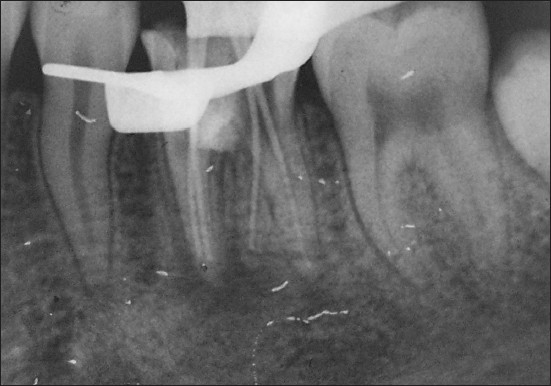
Master cone selection radiograph evidencing successful removal of separated instrument
